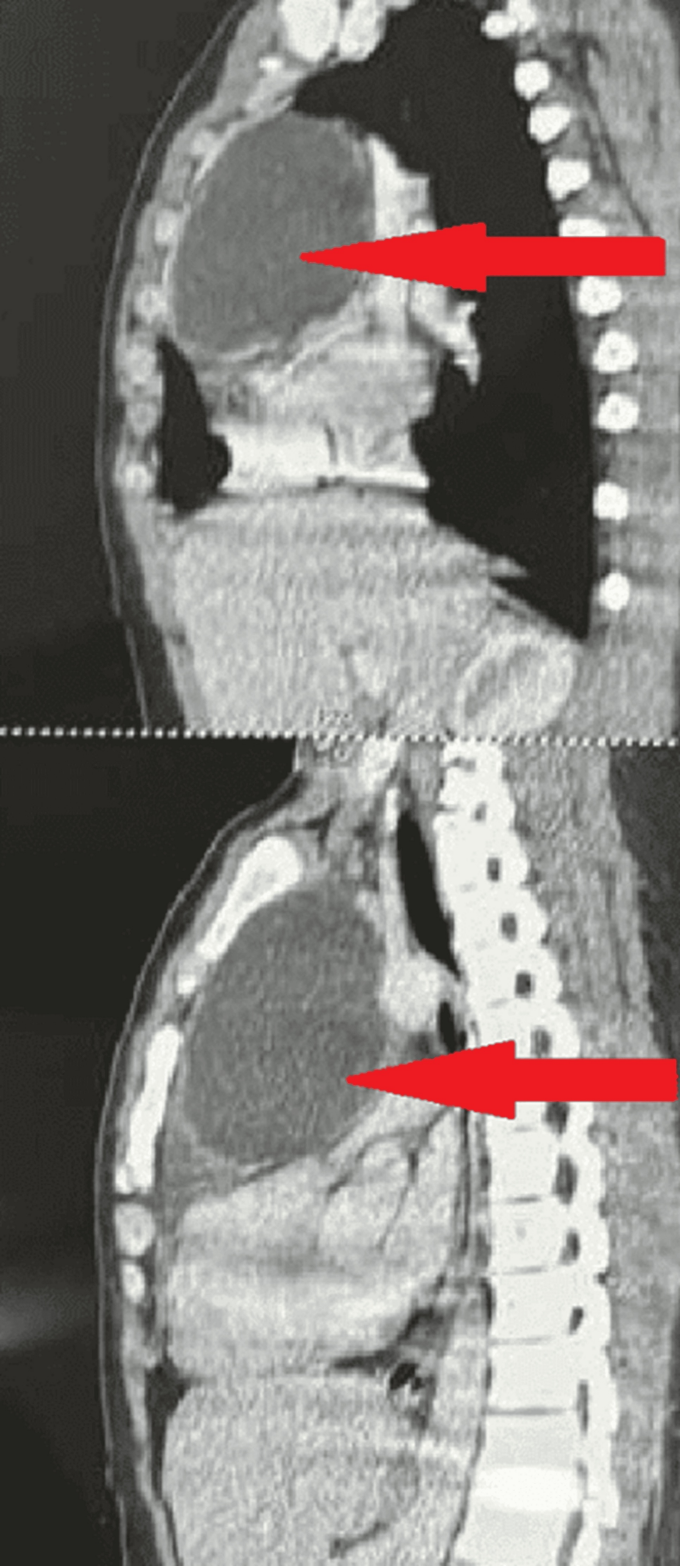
High-resolution computed tomography image of an anterior mediastinal mass
